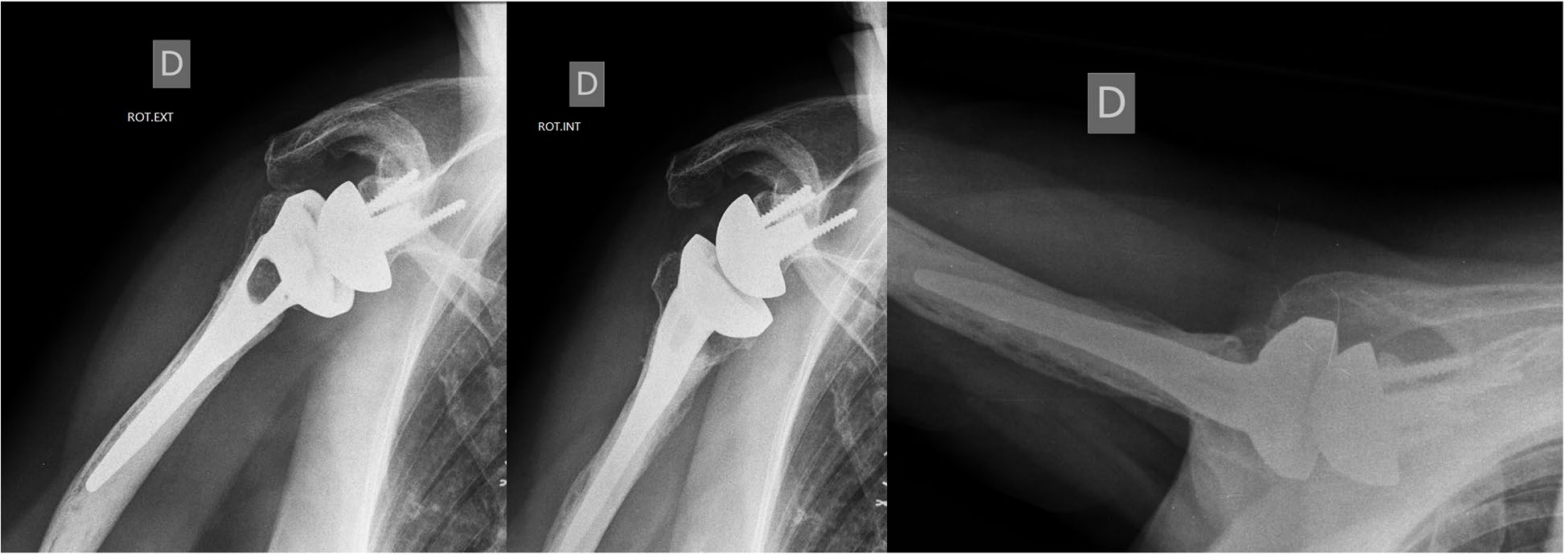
X-ray evaluation of the fracture-specific humeral stem (Aequalis Reversed-Fracture implant; Tornier, Memphis, TN, USA)
